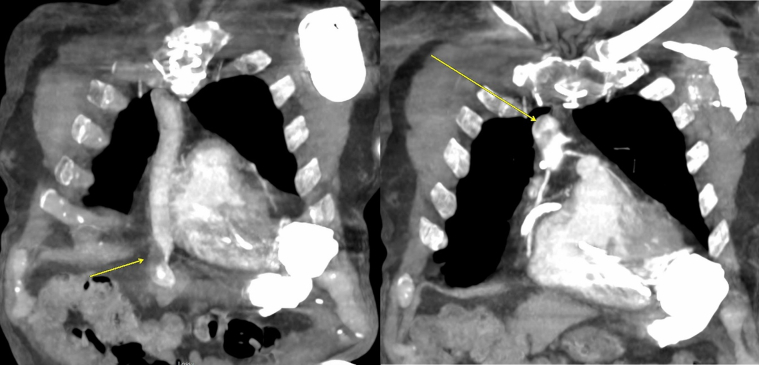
CTA Chest Images CTA chest demonstrating near-occlusive thrombus in LVAD outflow graft. CTA = computed tomography angiography; LVAD = left ventricular assist device.
